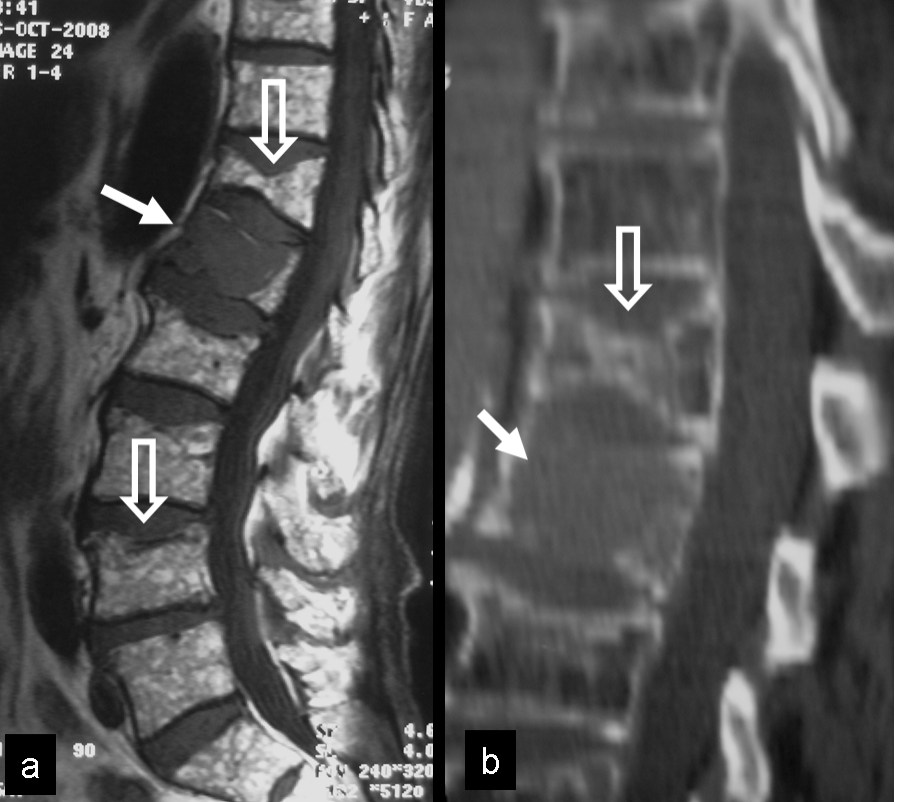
Diffuse thoracolumbar pain in a 71-year-old patient. The T1-w TSE MR image (a) and the sagittal reconstruction of the corresponding CT, showed diffuse infiltration of the L1 vertebral body (arrows). In addition, old osteoporotic fractures were noticed in the T12 and L4 vertebrae (open arrows). The bone marrow signal intensity on the MR image is inhomogenous.
